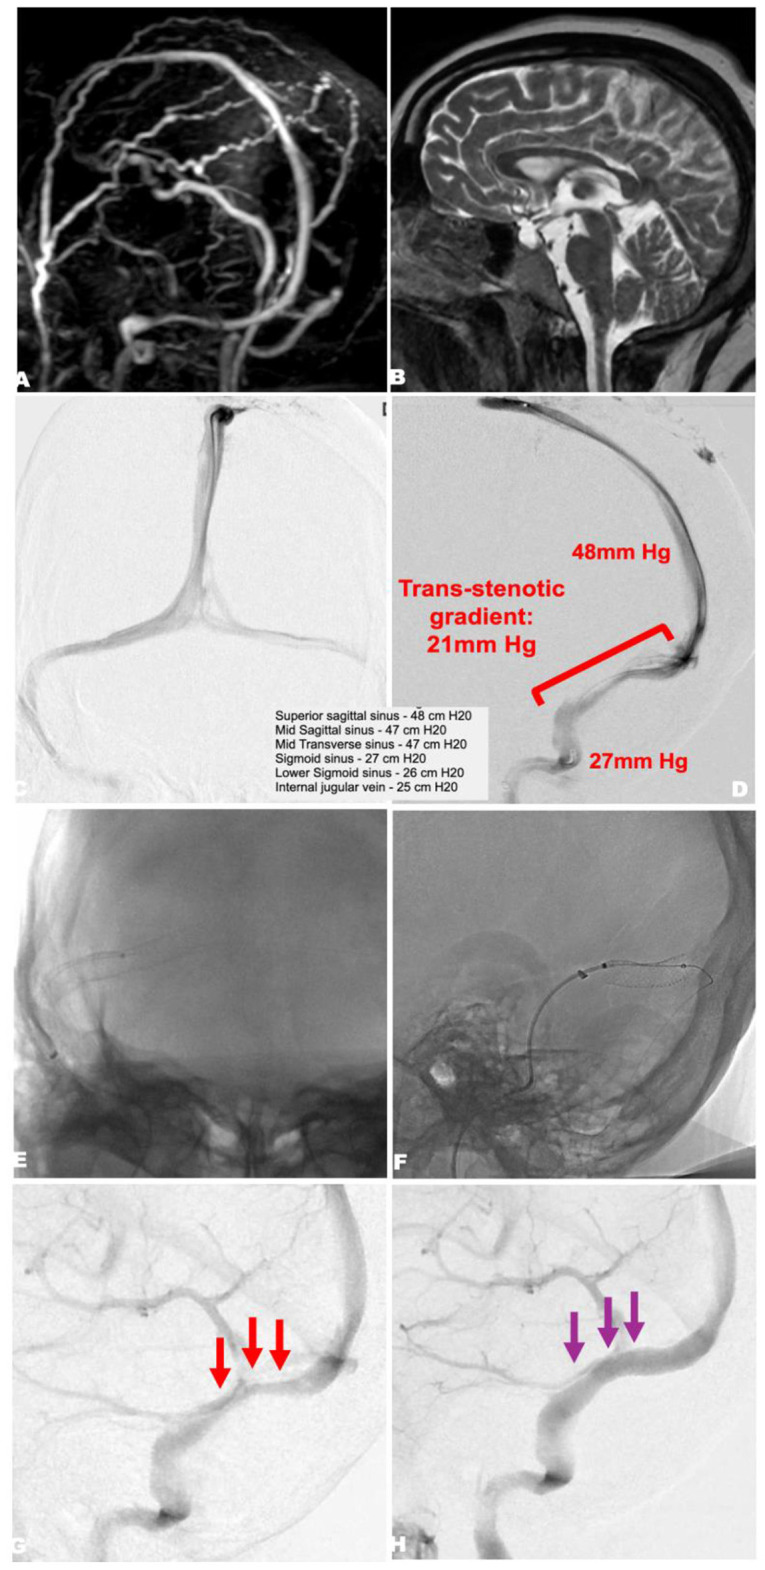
Urgent venous sinus stenting in fulminant idiopathic intracranial hypertension. A 36-year-old obese woman presented with 2 months of progressive vision loss, acutely worsening over 2 weeks. Ophthalmologic examination revealed severe bilateral papilledema (Frisen grade 4 OS, 4+ OD) with visual acuity 20/200 OS and light perception/finger counting OD. Lumbar puncture opening pressure was 46 cm H_2_O. She underwent single-session cerebral angiography, venography, manometry, and venous sinus stenting. (A) Time-of-flight MRA demonstrates focal stenosis of the right transverse sinus. (B) Sagittal T2-weighted MRI shows brain sag compatible with IIH. (C, D) Pre-stent venography and manometry confirm a right transverse–sigmoid sinus stenosis with a trans-stenotic pressure gradient of 21 mm Hg (48 → 27 mm Hg). (E, F) Deployment of a self-expanding venous stent across the stenosis from the transverse to sigmoid sinus. (G) Pre-stenting angiogram demonstrates flow restriction (red arrows). (H) Post-stenting angiogram shows restoration of normal sinus caliber and flow (purple arrows) with elimination of the pressure gradient.
